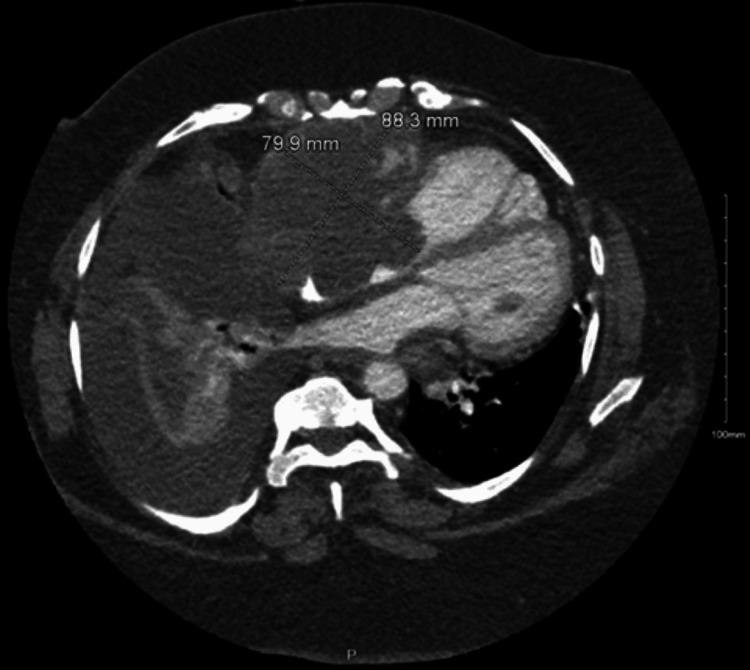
Chest CT showing a large lobulated mass in the right atrium measuring 8.8 cm x 8 cm.
